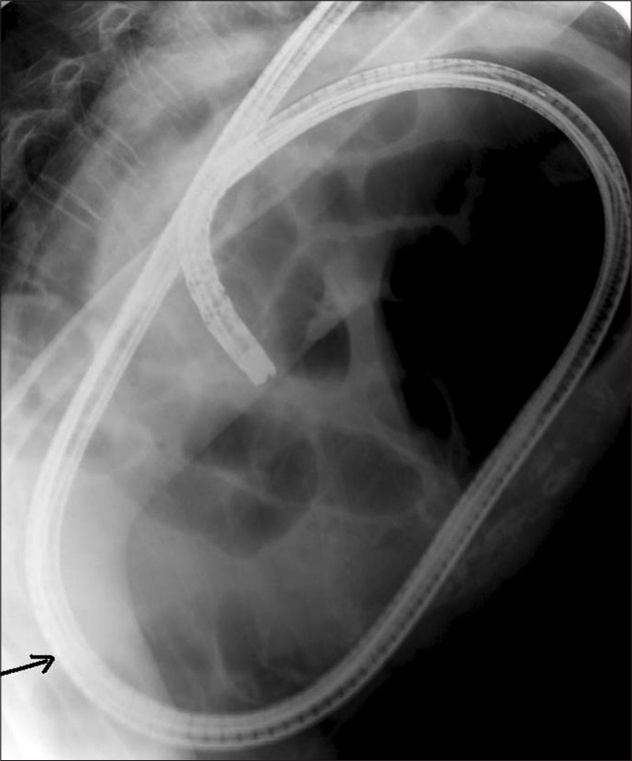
Lateral radiograph of the chest taken during endoscopy shows the scope to enter the air-fluid level within the right hemithorax (black arrow), suggesting right diaphragmatic hernia
